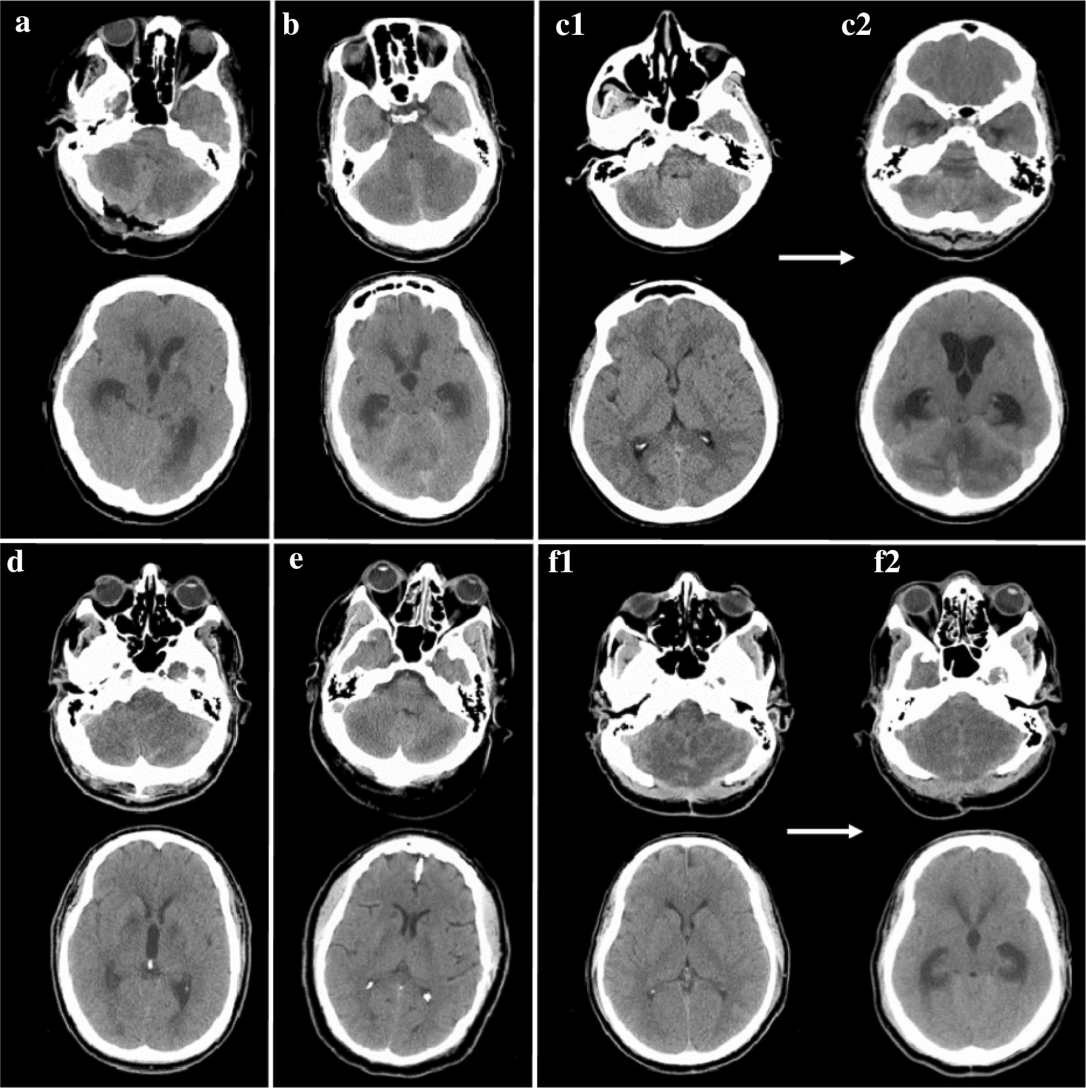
Non-contrast computed tomography of the head from patients at the time of arrival or transfer to tertiary care center, at the level of the cerebellum (above) and the lateral ventricles (below). A Patient #1, CT on arrival to tertiary care center, 3 days after initial event; emergent suboccipital decompression was completed on arrival; B Patient #2, at time of presentation, ~ 48 h after last well; C1 Patient #3, on arrival; C2 Patient #3 on hospital day 6, demonstrating evolution of cerebellar edema with obstructive hydrocephalus; D Patient #4, at time of presentation, ~ 24 h from last well; E Patient #5, at time of presentation, ~ 24 h from last well; F1 Patient #4, at time of presentation, ~ 48 h from last well; suboccipital decompression is from a previous event; F2 Patient #4 on hospital day 4, demonstrating evolution of cerebellar edema with obstructive hydrocephalus
